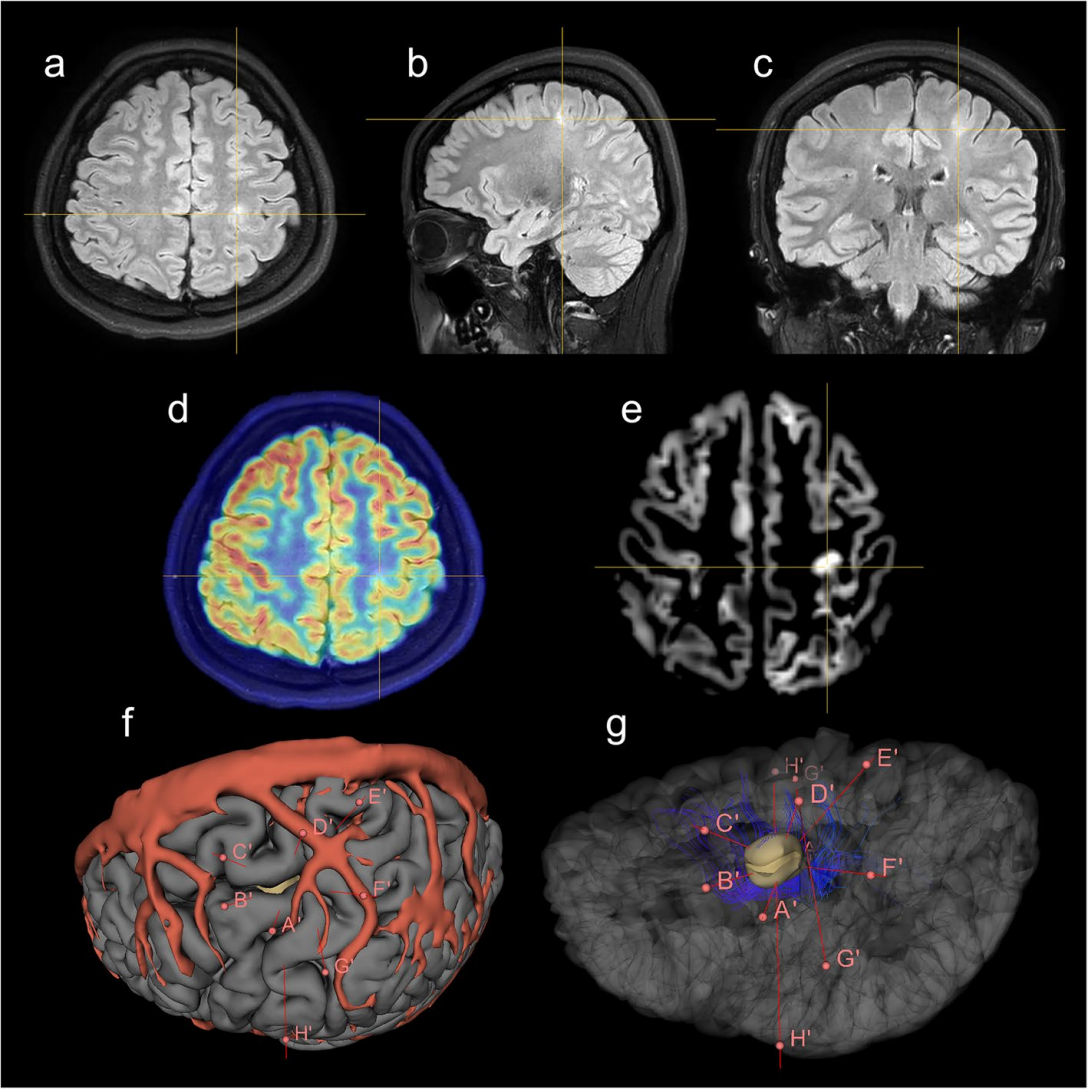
Representative images of electrode implantation designed with image reconstruction (Patient 6). a–c Preoperative MRI FLAIR images in the axial, sagittal and coronal plane with the FCD in the left precentral gyrus. d Fused image of MRI FLAIR and PET with the FCD. e Segmentation of gray matters based on MRI T1 images with the FCD. f–g Reconstruction model of the cerebral surface (gray), vessels (red), electrodes (red lines, with corresponding letters of electrodes), FCD structure (yellow) and corticospinal tract (blue lines), with the personalized SEEG electrode-implantation trajectories based on the models. Crossing point of yellow lines in (a–e) is the location of the FCD in the left precentral gyrus. Abbreviation: SEEG, stereo-electroencephalography; FCD, focal cortical dysplasia
